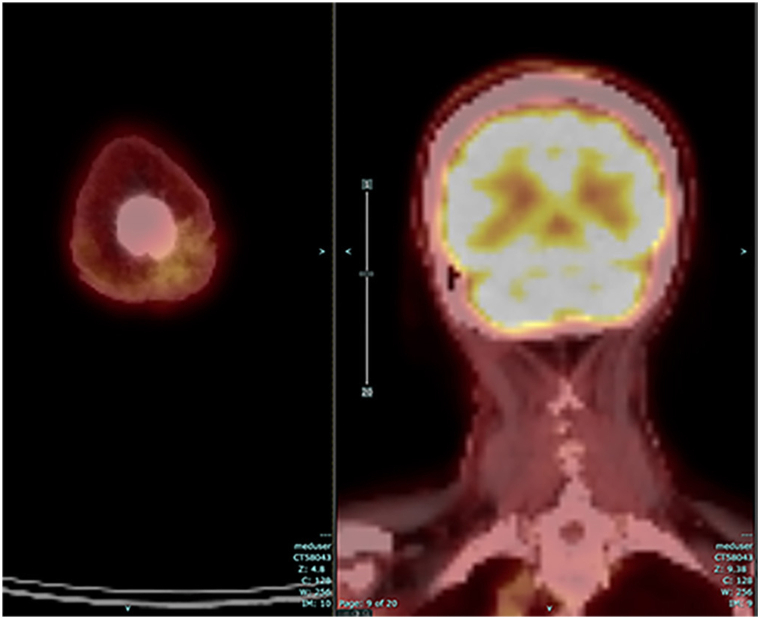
Fused FDG PET-CT axial and coronal images after 5 cycles of anti–PD-1 therapy; size of scalp lesion decreased from 2.9 cm × 4.0 cm to 2.2 × 3.6 cm (and less dense), and SUV_max_ decreased from 13 to 2.6, compatible with a favorable response to treatment. *FDG*, fluorodeoxyglucose; *PD-1*, programmed death-1; *PET-CT*, positron emission tomography-computed tomography; *SUV*_*max*_, maximum standardized uptake value.
